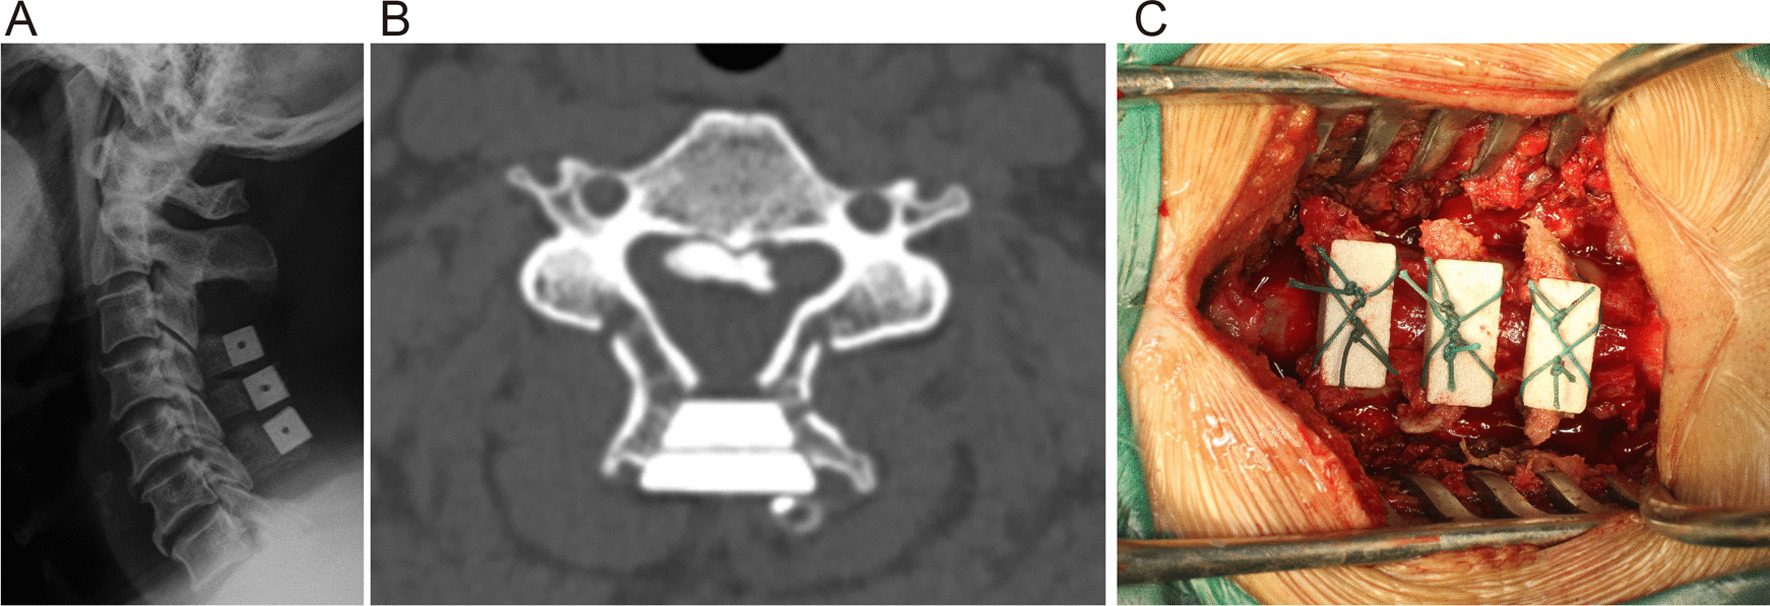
Lateral X-ray and axial computed tomography slice of patients with C-OPLL undergoing mFDL. A and B The laminas were opened from both sides, and the split spinous processes were fused with coralline hydroxyapatite (CHA) spacers. C Operative picture of mFDL, including laminectomy of C3 and the upper half of C7 as well as fixation between the spinous processes and CHA spacers (C4–6) using silk thread
